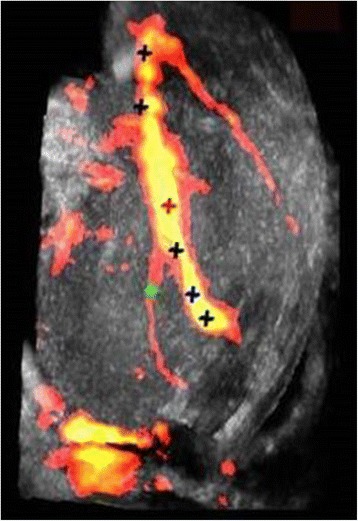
Image of limb vascularity in a test subject with SFA bleeder branch. Compound 3D power Doppler image with D&L automated algorithm-placed markers (*crosses*) denoting normal branches (bifurcations), vascular centerline markers, and the bleeder target (*green cross*), here 10 mm distal to the bleeder SFA branching point
